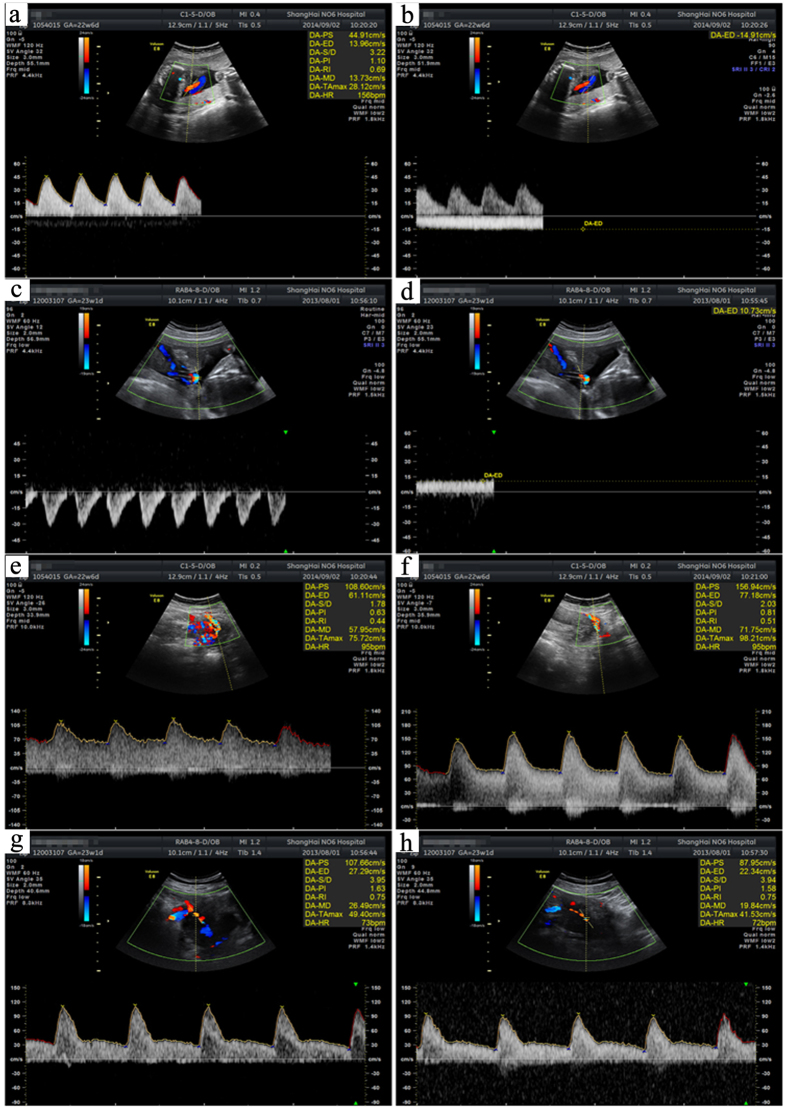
Representative Doppler ultrasound images at ~23 weeks of pregnancy. (a) Normal pregnancy umbilical artery. (b) Normal pregnancy umbilical vein. (c) HRHypP (umbilical artery with fetal death. (d) HRHypP umbilical vein with fetal death. (e) Normal pregnancy right uterine artery. (f) Normal pregnancy left uterine artery. (g) HRHypP right uterine artery with fetal death. (h) HRHypP uterine artery with fetal death.
